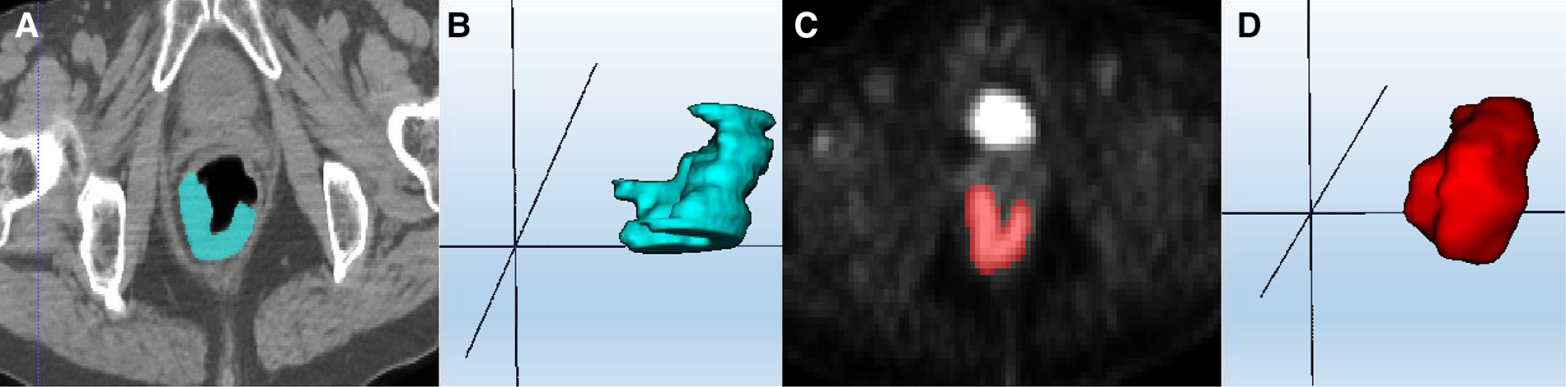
ROI segmentation of colorectal cancer lesions. (A) CT ROI segmentation; (C) PET ROI segmentation; (B,D) VOI based on multiple single-layer ROI fusion.
